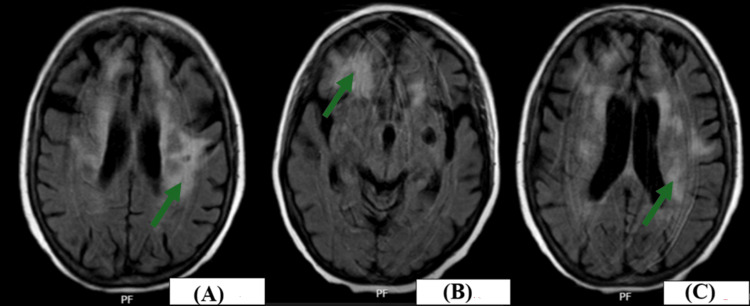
Confluent white matter lesions throughout all hemispheres seen on MRI brain FLAIR imaging (A,C) Left-sided cerebral periventricular white matter lesions (green arrows). (B) A right-sided frontal cortex white matter lesion (green arrow) MRI: magnetic resonance imaging; FLAIR: fluid-attenuated inversion recovery
